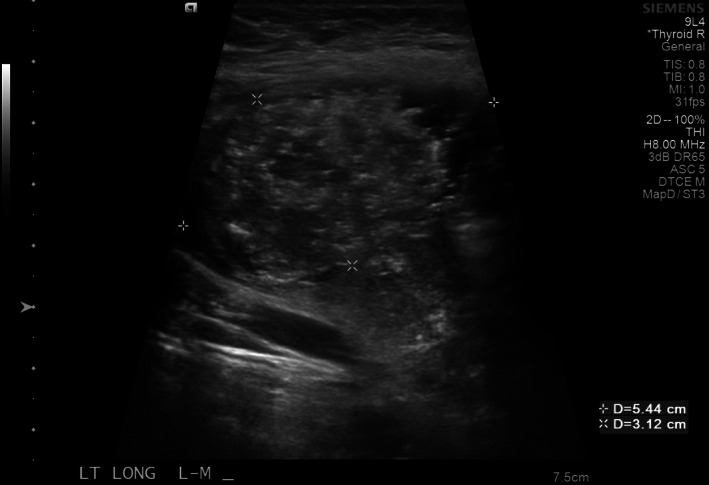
Thyroid ultrasound demonstrating multiple bilateral nodules concerning for malignancy. Pictured below is a TI‐RADS 5, left‐sided thyroid nodule, approximately 5.4 cm in greatest dimension
